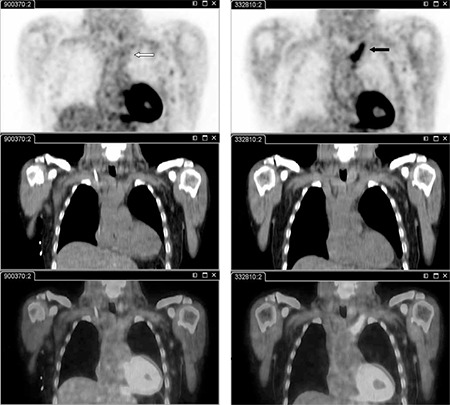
FDG-PET/CT in the diagnosis and treatment evaluation of deep septic thrombophlebitis. Coronal PET, CT and fused PET/CT images (low dose CT used for attenuation correction and localization). Original PET/CT scan (black arrow) and the follow up PET/CT 4 weeks later (white arrow). The activity in the brachiocephalic vein that is seen on the initial PET (black arrow) cannot be found on the follow up PET scan (white arrow).
